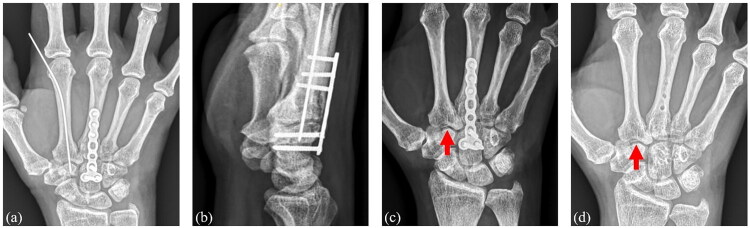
(a, b) Radiographs show retrograde intramedullary fixation of the reduced trapezoid–metacarpal joint and a spanning plate for the middle finger metacarpal base fracture at 1 month postoperatively. Radiographs at 3 months (c) and 1 year (d) postoperatively show a maintained reduction of the trapezoid–metacarpal joint (red arrow).
